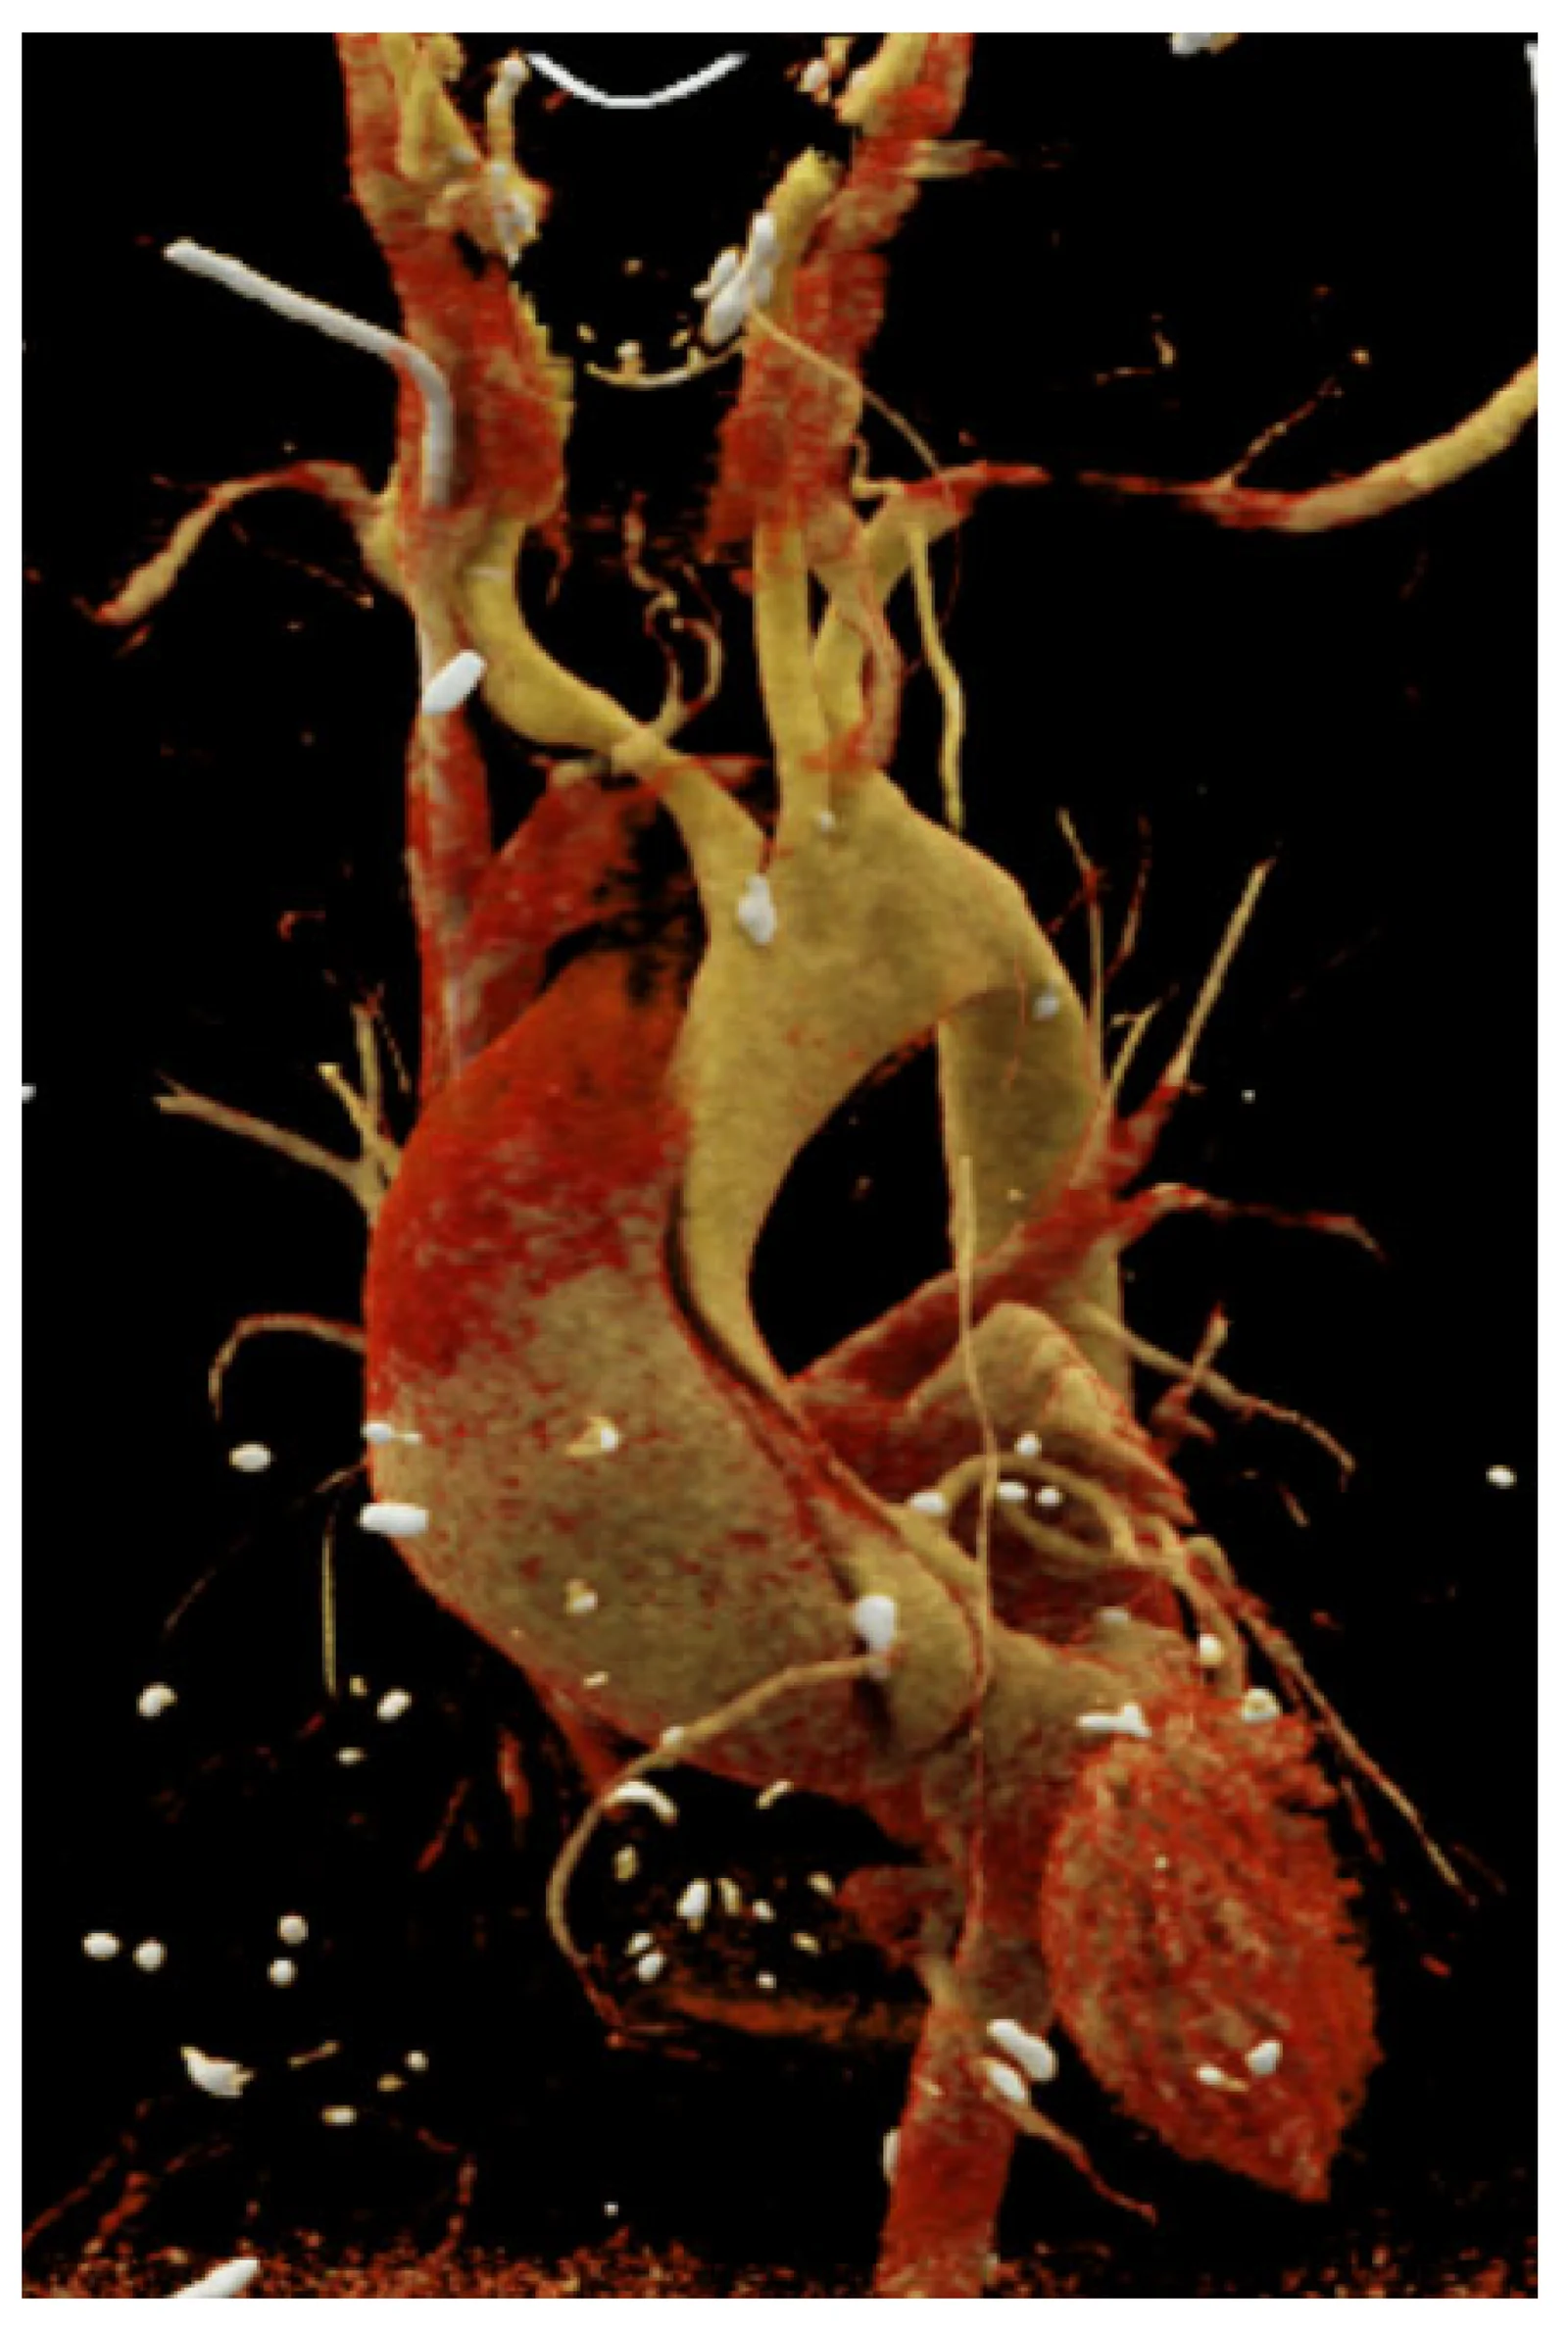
Reconstruction view of the Stanford type A aortic dissection. Three-dimensional reconstruction of computed tomography angiography demonstrating the acute Stanford type A aortic dissection.
